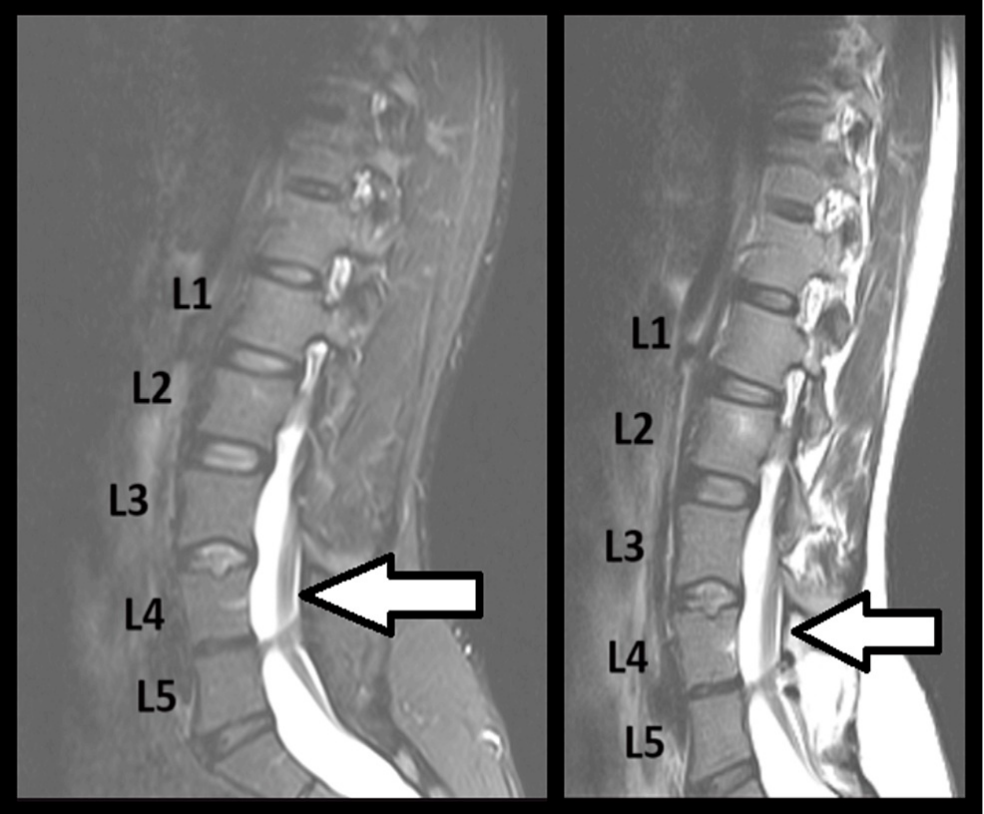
MRI of L4-L5 diastematomyelia (sagittal plane) The hemicords have been split by a bony spur at the level L4-L5. Deformations of L4 and L5 vertebrae are visible. Note the bony spur dividing the hemicords (arrows).
